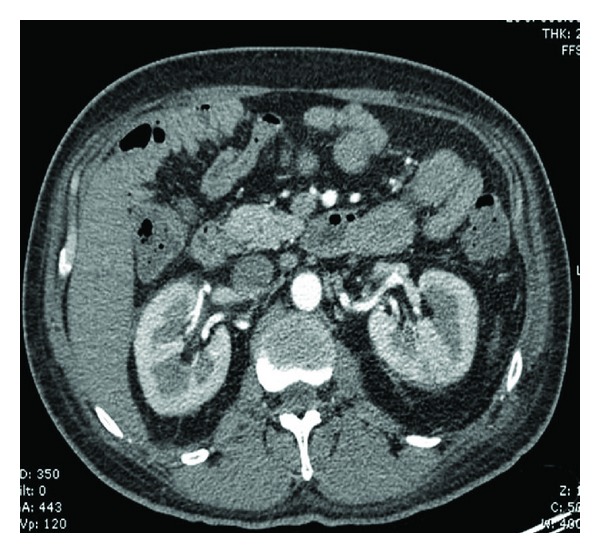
CT angiogram with no thrombus after 1 week of treatment.
